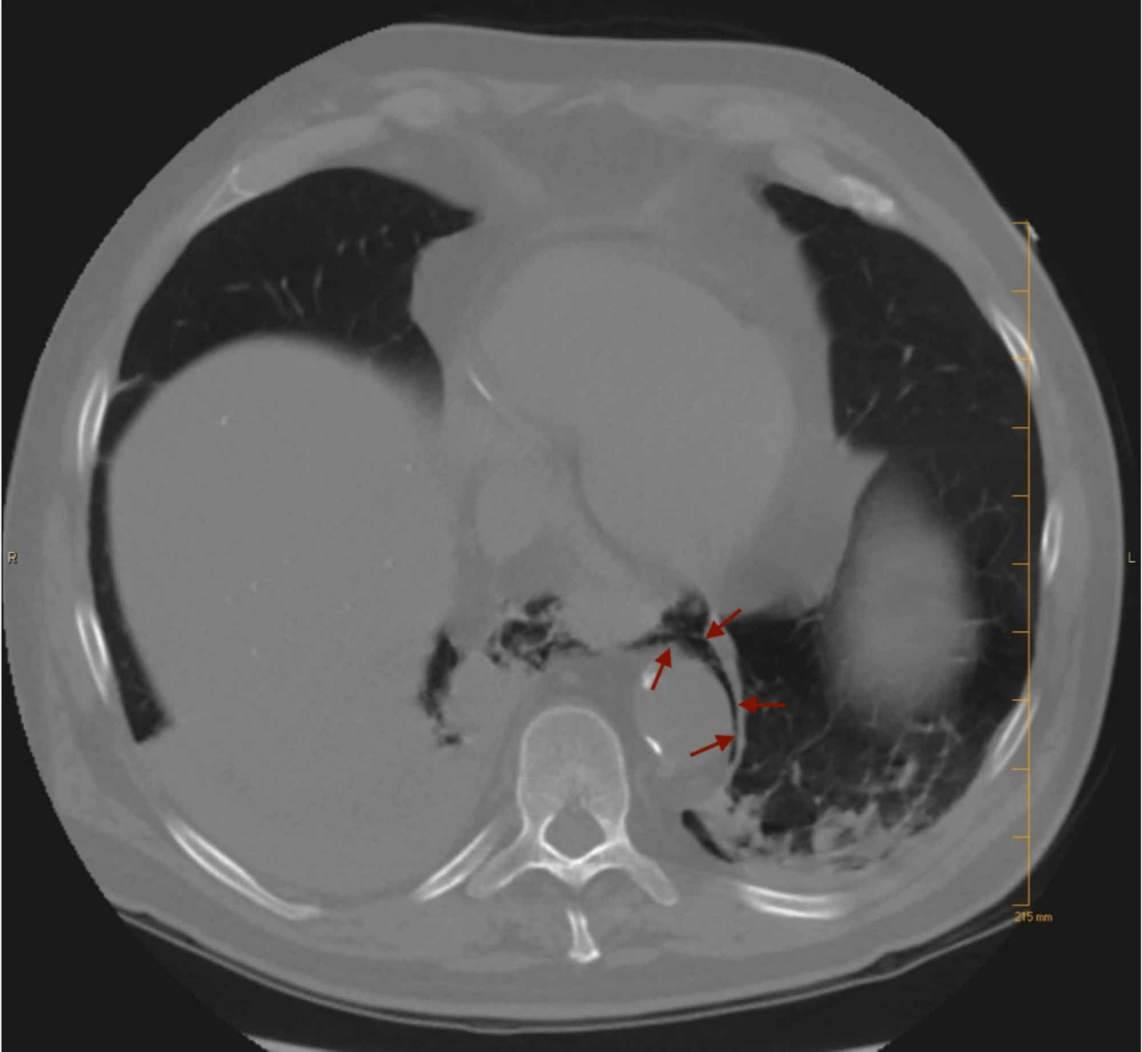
CT of the chest showing pneumomediastinum
